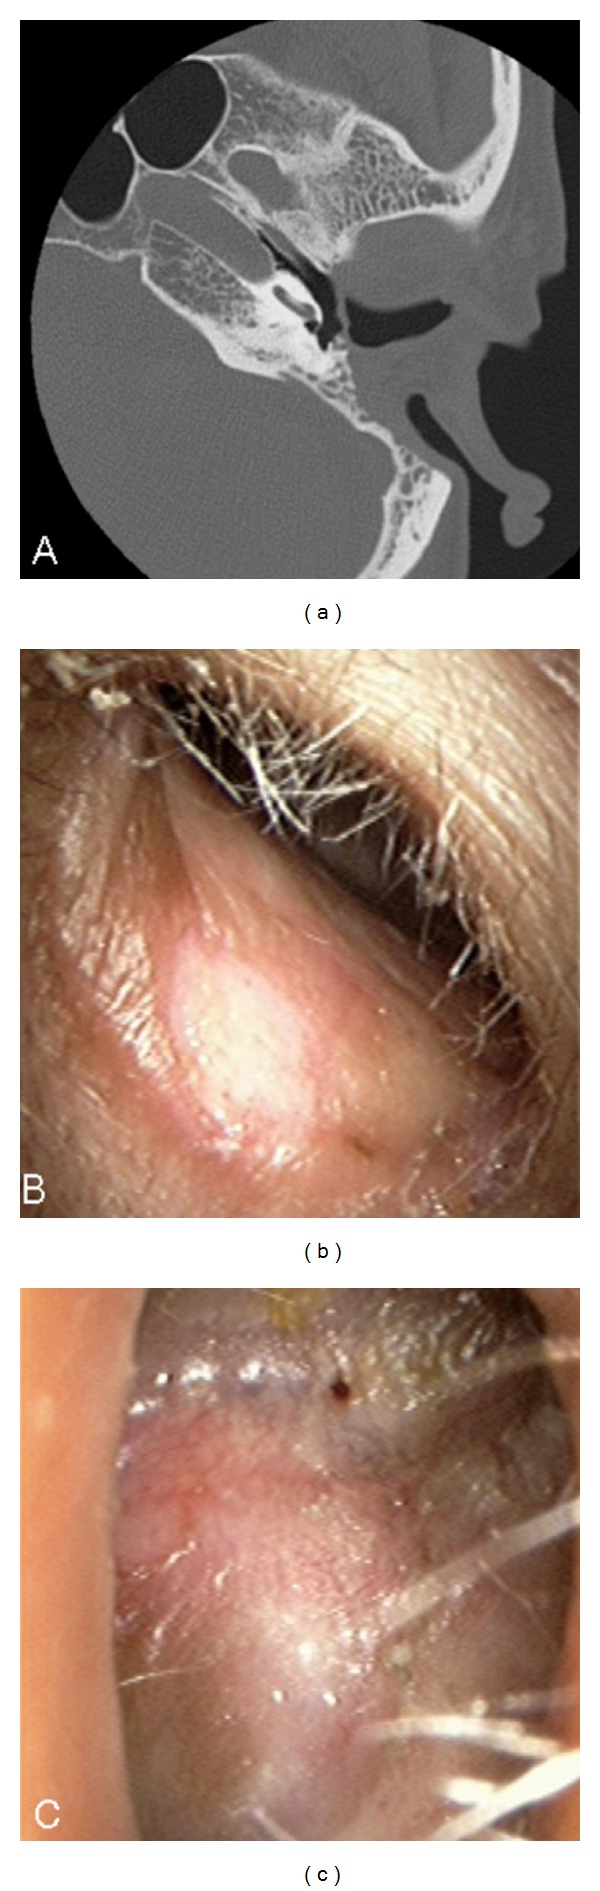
One-year postoperative findings in Case 2. (a) An axial CT image showed that the reconstructed EAC was kept in good shape, and no lateralization of the tympanic membrane was observed. (b and c) No stenosis or infection of the reconstructed external auditory canal was observed.
